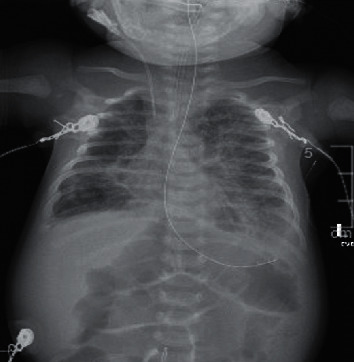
Initial chest X-ray showing bilateral pulmonary edema with left pleural effusion.
